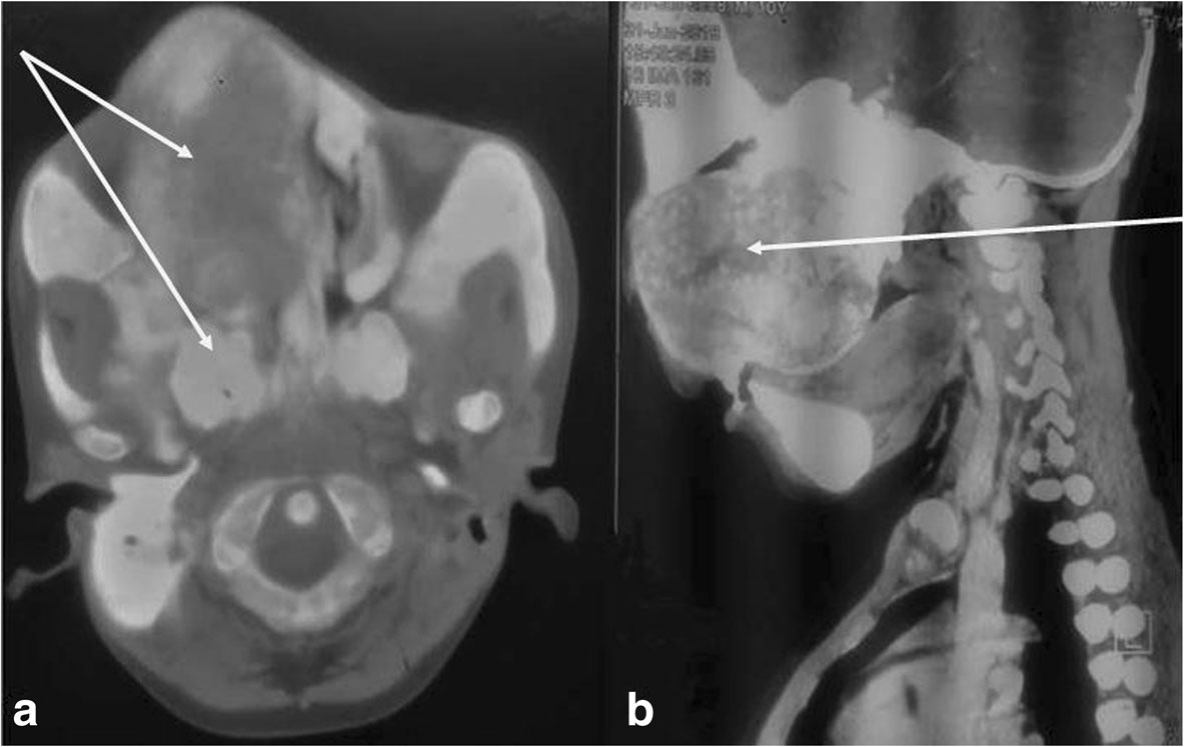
a & b: CT-scan (axial and sagittal cuts) showing a huge midfacial lesion which has a ground glass appearance involving the maxilla, palate, zygomatic bone, frontal bone and base of the skull. Both maxillary sinuses are obliterated. The lesion is filling the oral cavity
